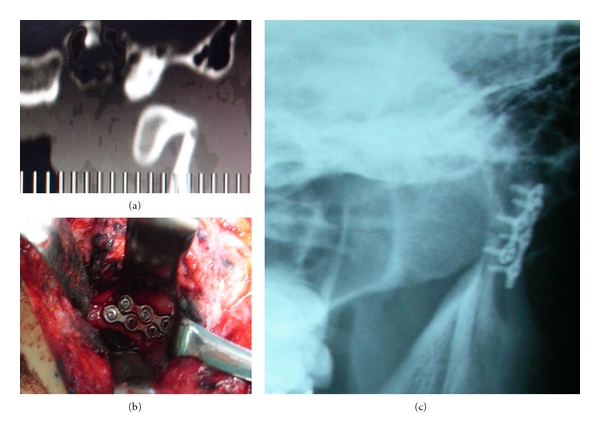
(a) CT shows a fracture of the left subcondyle with the condylar head dislocated anteromedially. (b) The condylar head was reposited, and two miniplates were fixed across the fractured line. (c) Postoperative radiography, showing reduction of the fracture segment.
